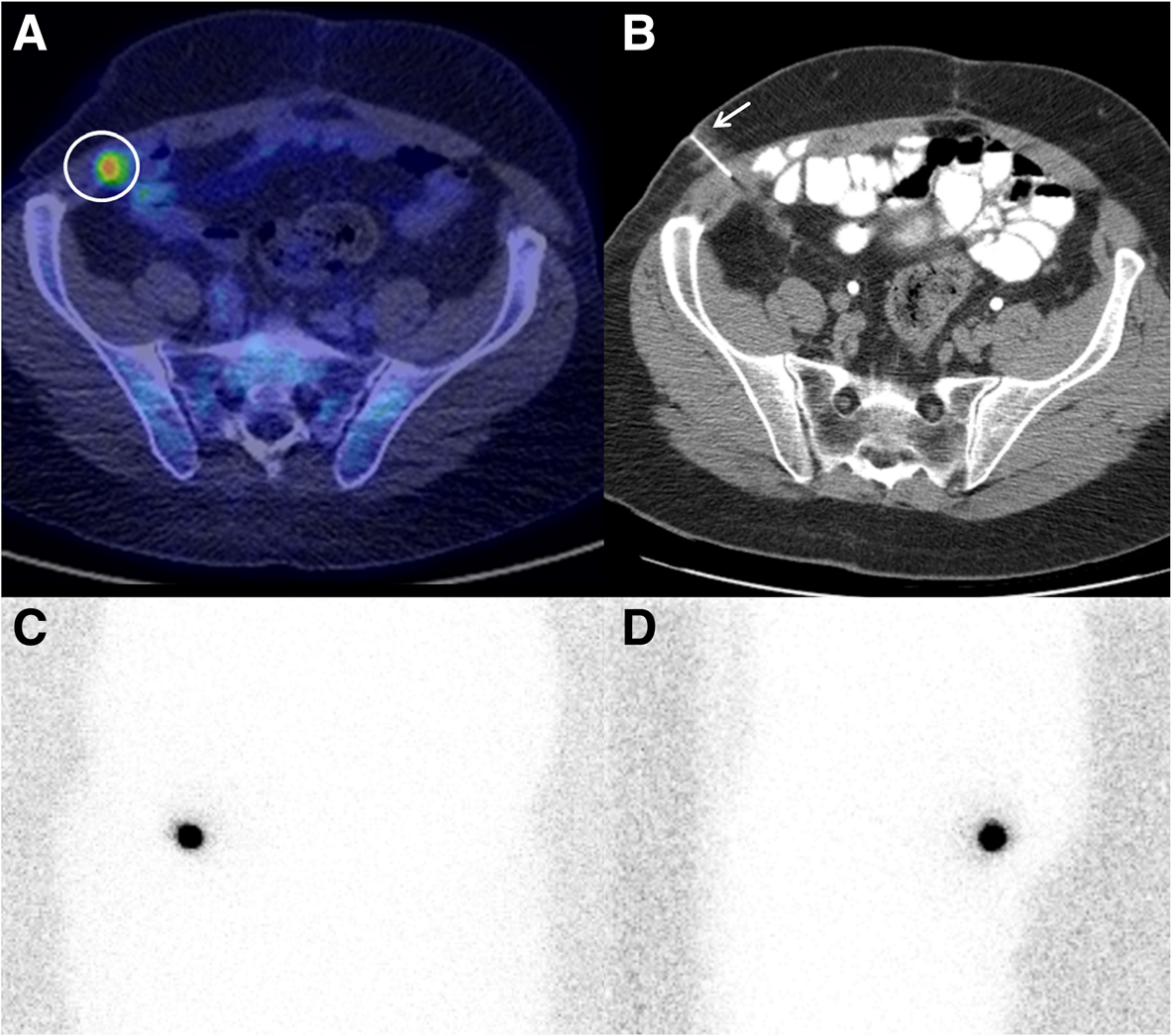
A 66-year-old male with right colon adenocarcinoma metastatic to the liver, status post right hemicolectomy, hepatic wedge resection, and chemotherapy. A follow-up FDG PET/CT (a) demonstrated a hypermetabolic nodule in the right lower quadrant abdominal wall (white circle) which was non-palpable. CT-guided (b) Tc-99m MAA was injected (white arrow) in the region of this hypermetabolic nodule. Anterior (c) and right lateral (d) scintigraphic views of the abdomen demonstrate excellent localization. This lesion was surgically resected and positive for metastatic adenocarcinoma
